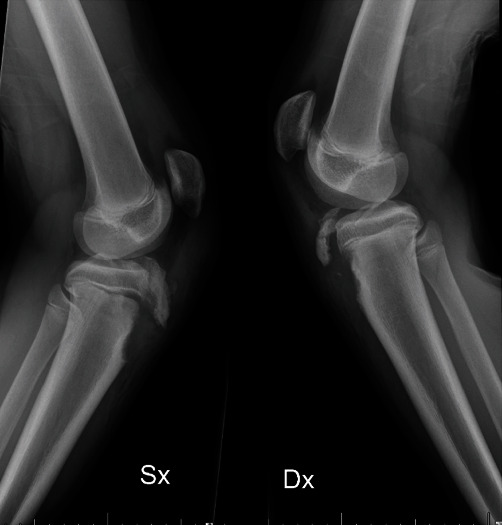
Case I: X-ray imaging showing asymmetrical bilateral tibial tuberosity avulsions with a type IIB of the right knee and type III B of the left, without intra-articular involvement.
